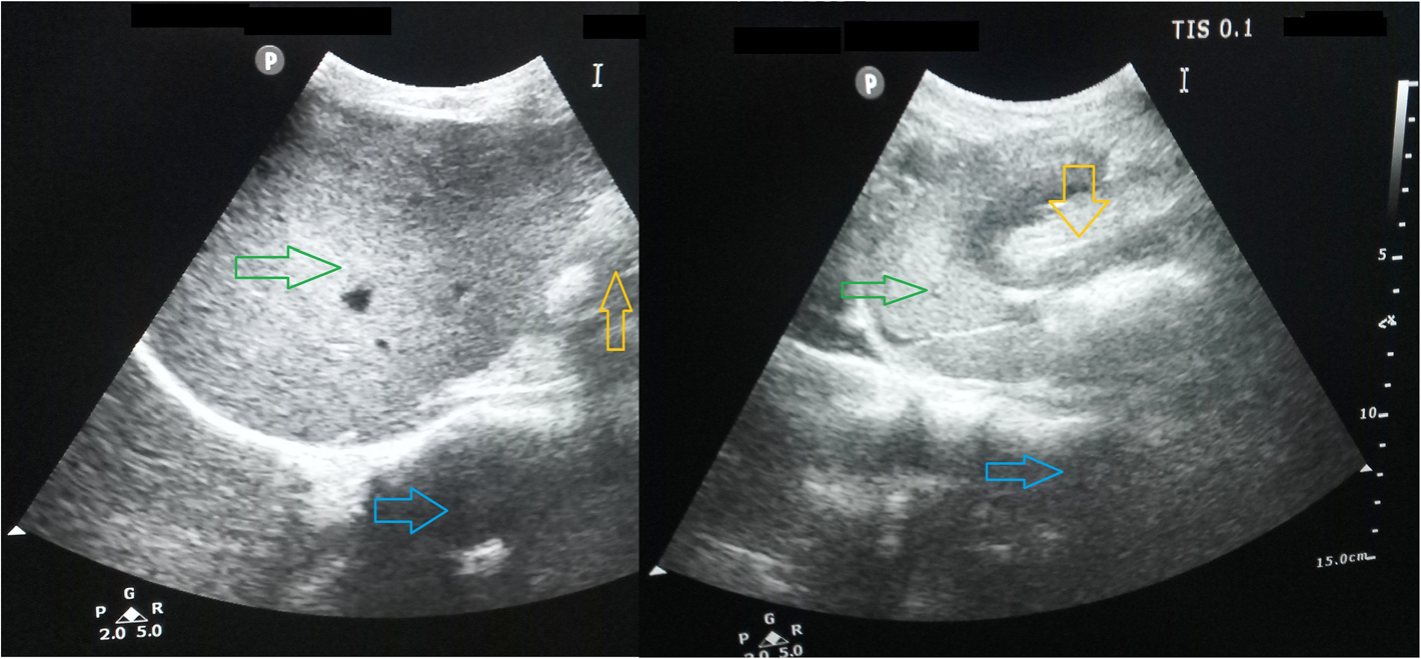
Post-operative Day 3 greyscale Ultrasonography of the liver (Green arrows) and epigastric region revealed the position of hypo-echoic omental fat (Yellow arrows) that was placed below the left lobe of the liver during operation and position of the pancreas (Blue arrows).USG shows no demonstrable collection in the operative field
